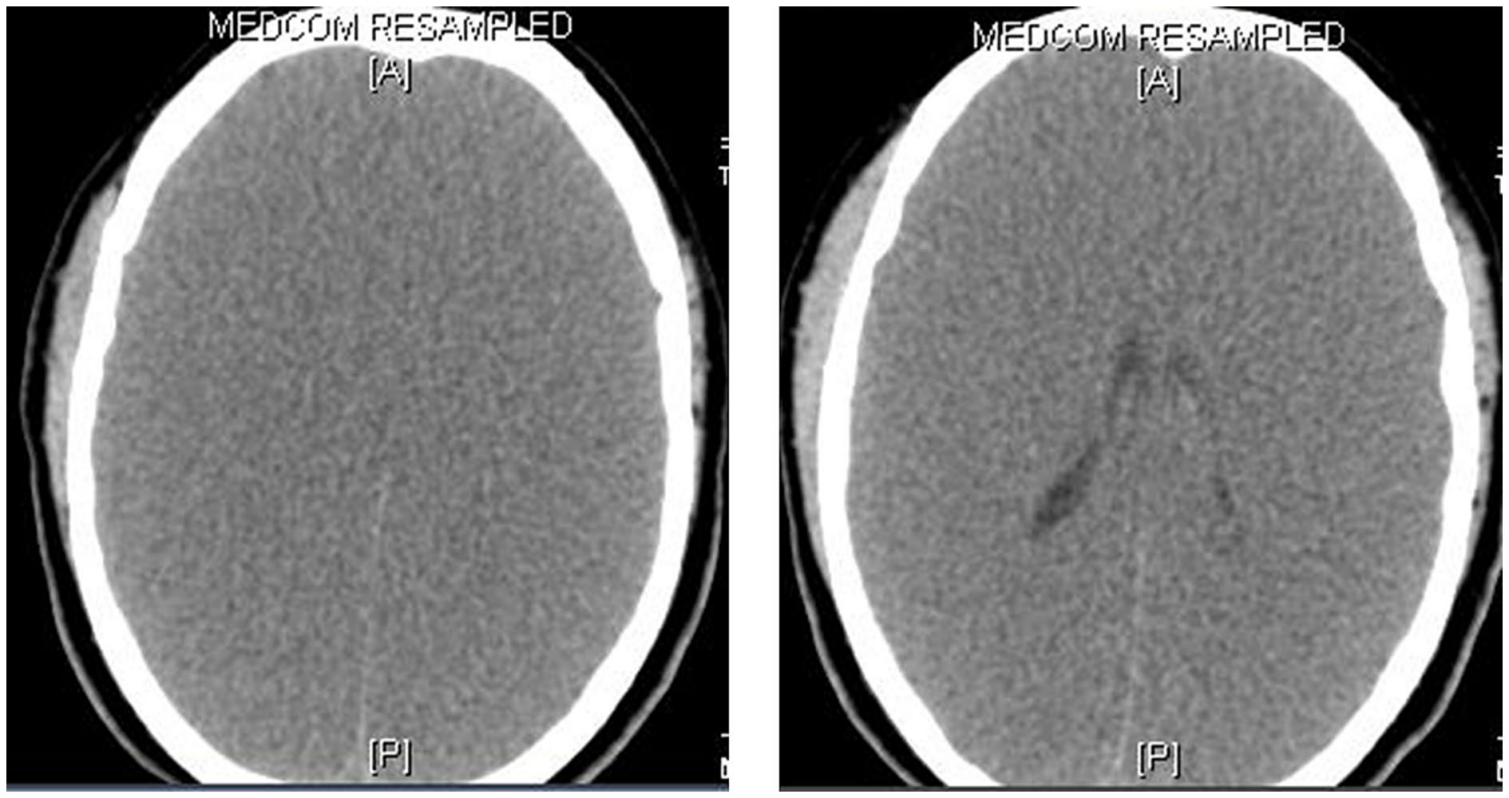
CT scan of the brain in brain death. This 8-year-old boy presented with a rapidly deteriorating level of consciousness and generalized seizures for 2 days. He was diagnosed with viral encephalitis. While on mechanical ventilation, his pupils were noted to be fixed and dilated on day 4 and there were no elicitable brainstem reflexes. The CT scan of the brain showed generalized cerebral edema, loss of the gray-white matter junctions, and effacement of the sulci, ventricles, and basal cisterns.
